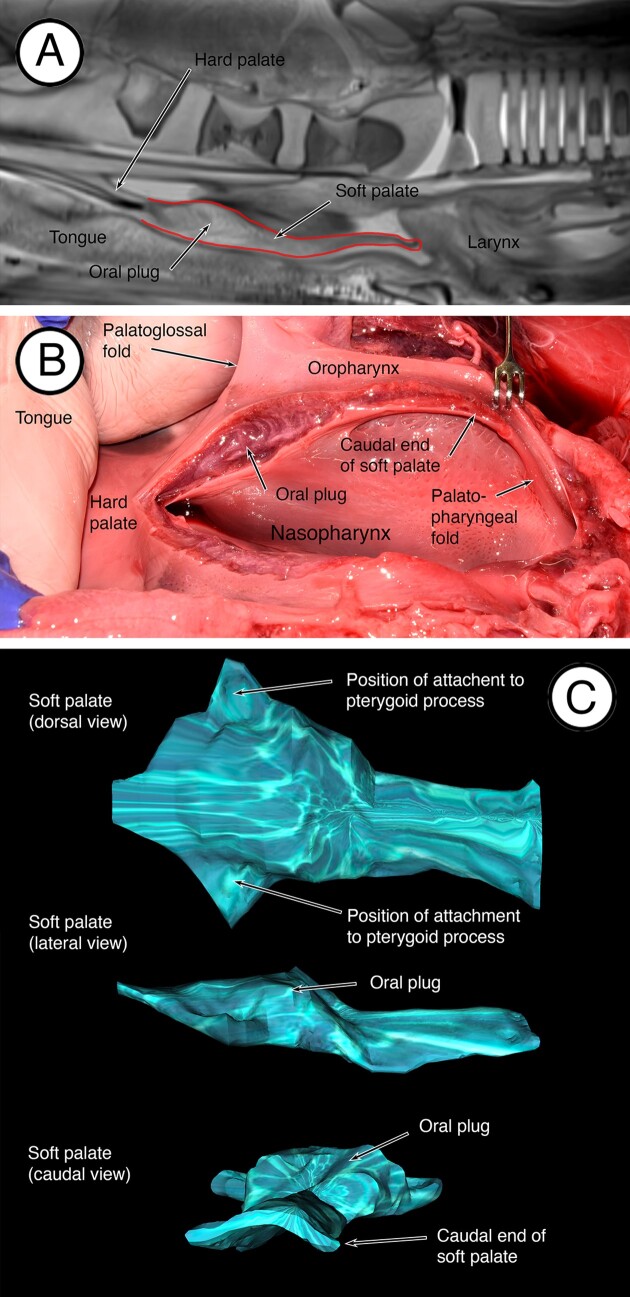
Developing soft palate in fetal fin whales. (A) MRI scan in the mid-median plane showing the soft palate (outlined) with the developing oral plug at the rostral end. The rostral end of the larynx is positioned just above the caudal free edge of the soft palate. (B) In this dissection, the fetus is on its back. The soft palate has been sectioned in the mid-median to show the developing oral plug at the rostral end. The palatopharyngeal fold merging with the pharyngeal wall is evident at the caudal end of the soft palate. (C) Reconstructions of the soft palate created from the MRI dataset using OsiriX MD software.
